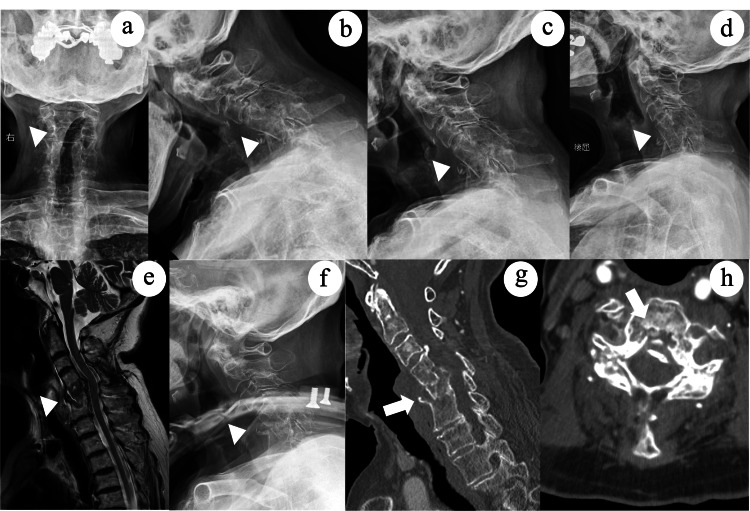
Radiological findings before surgery. (a-d) Plain radiographs of the cervical spine showing a flexion deformity fracture of the fused vertebrae and dynamic instability (arrowheads). (e) Magnetic resonance imaging showing mild spinal cord compression at the fracture site (arrowhead). (f) Lateral radiograph of the cervical spine after halo fixation showing stabilization at the fracture site (arrowhead). (g) Sagittal and (h) axial computed tomography scans reveal mild stenosis at the fracture site caused by posterior wall fragments (arrows).
